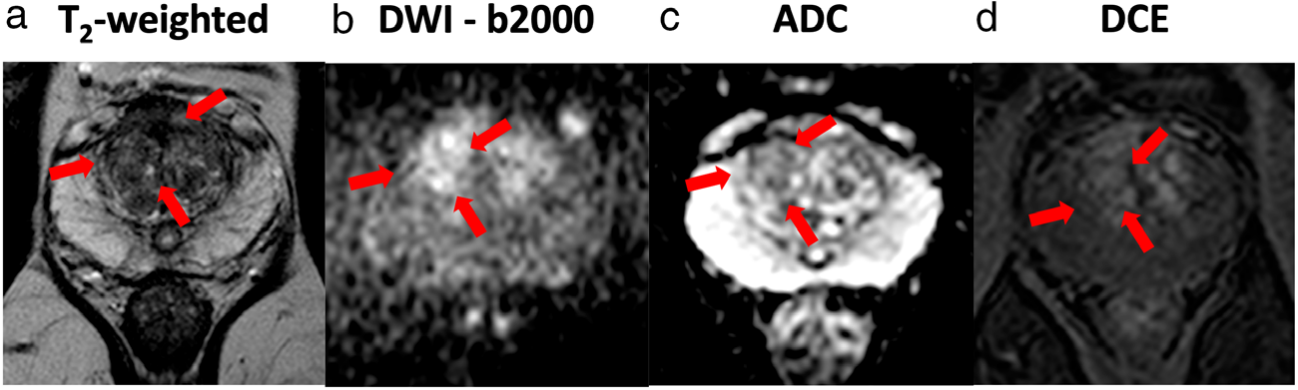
Example of the mpMRI data from a subject with PCa. (Left to right) (a) T2‐weighted image (T2W), (b) diffusion‐weighted Image (DWI)—b2000, (c) apparent diffusion coefficient (ADC) map and (d) dynamic contrast‐enhanced MRI (DCE). In each image, the tumor location is outlined with red arrows.
